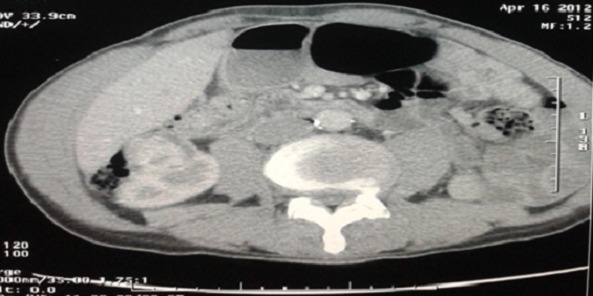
Evaluation after 2 cycles of chemotherapy with a CT scan of the abdomen. CT scan cut showing a stability of the renal mass according to RECIST 1.1
